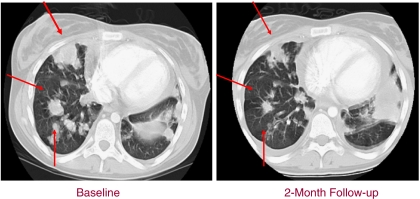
CT scan of thoracic cavity of a patient with refractory HD before and after i.v. vorinostat 600 mg m^−2^ for 5 days every 3 weeks.
